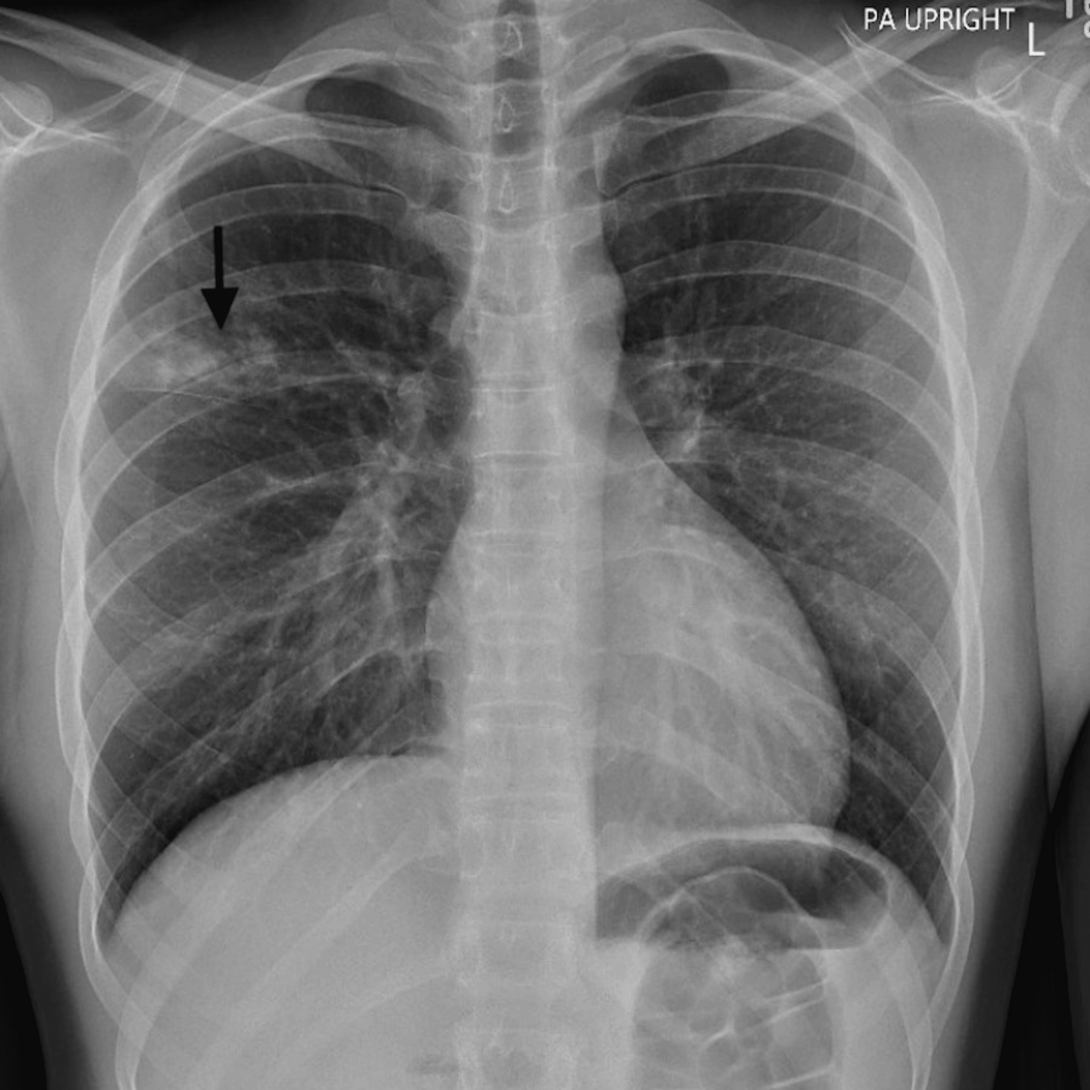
Chest X-ray at presentation showing opacity in the right upper lobe consistent with pneumonia
